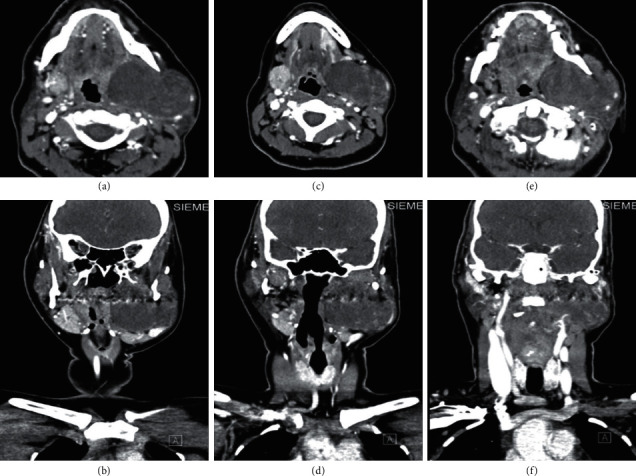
(a–c) Axial cut CT images showing the heterogenous left-sided smooth surface mass with minimum mass effect on the oropharyngeal airway, with no destruction of the mass and no intracranial extension. Mass appears to be around 10 cm in size. (d–f) Coronal cut CT images showing the mass from the left side with no mass effect on oropharyngeal airway.
